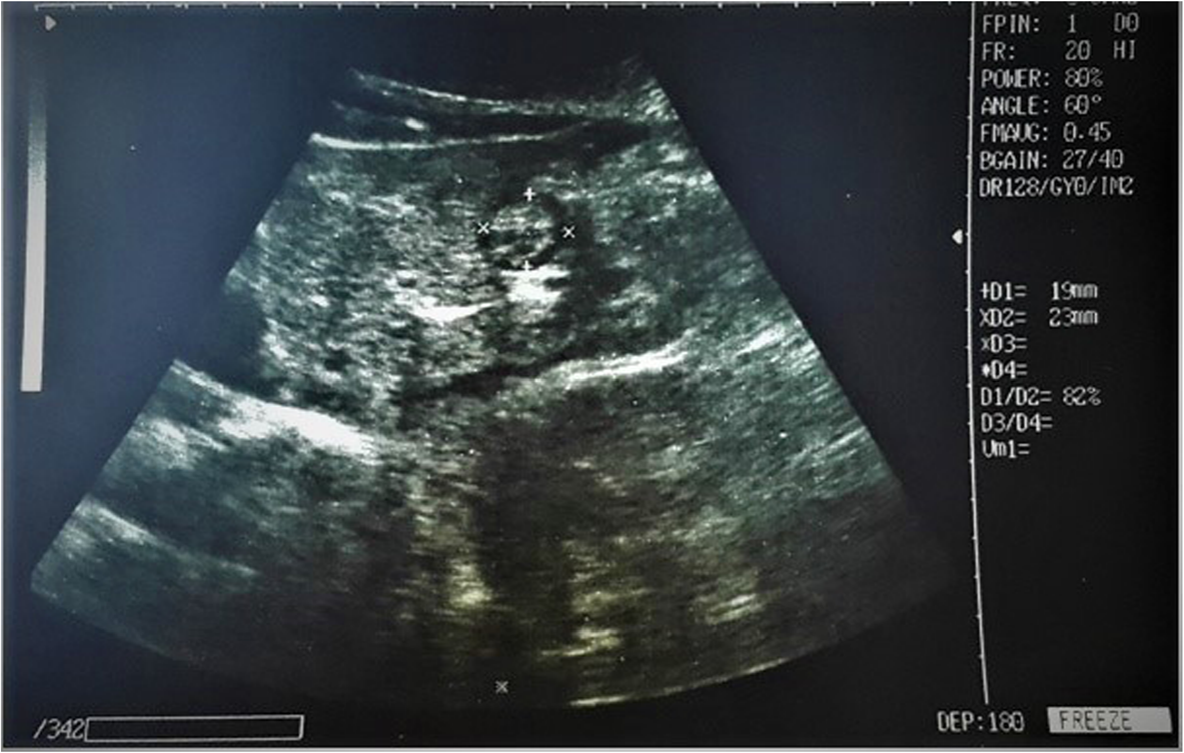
Ultrasound image of the gastric antrum (epigastric region) in the parasagittal plane, obtained after 8 h fasting in the preoperative period
